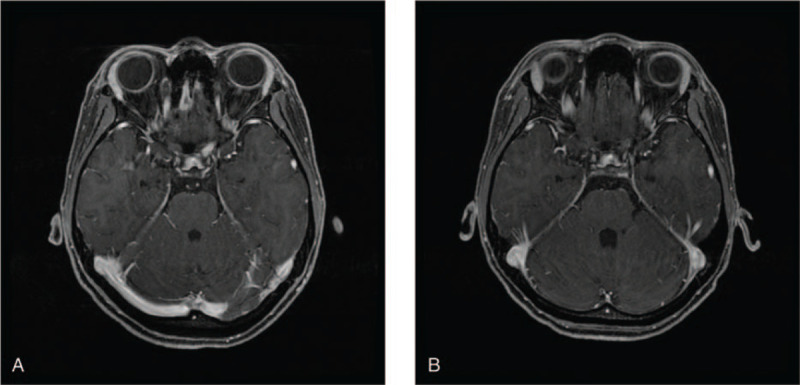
(A). On day 3 of crizotinib therapy, brain MRI shows that the enlargement of left optic nerve. (B). After 4 doses of IT and WBRT (14.4Gy), brain MRI reveals an improvement of optic nerve enlargement.
